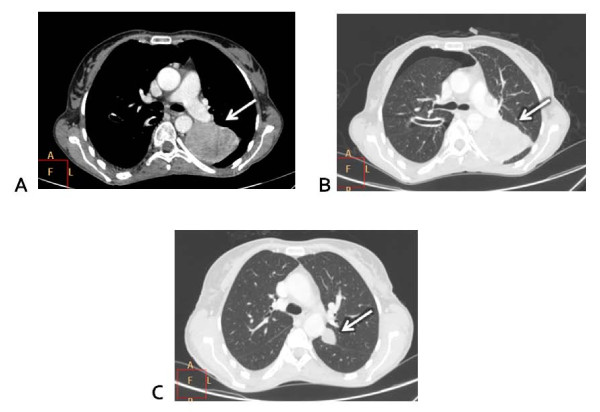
Computed tomography scan evaluation. (A) Baseline: great metastatic nodular lesion on the left lung (white arrow). (B) Baseline: lung parenchymal windows showing the great lesion on the left and a drainage tube for thoracentesis with residual pneumothorax (white arrow). (C) Week 12 under treatment: reduction of the great lung lesion with small residual nodule (white arrow).
